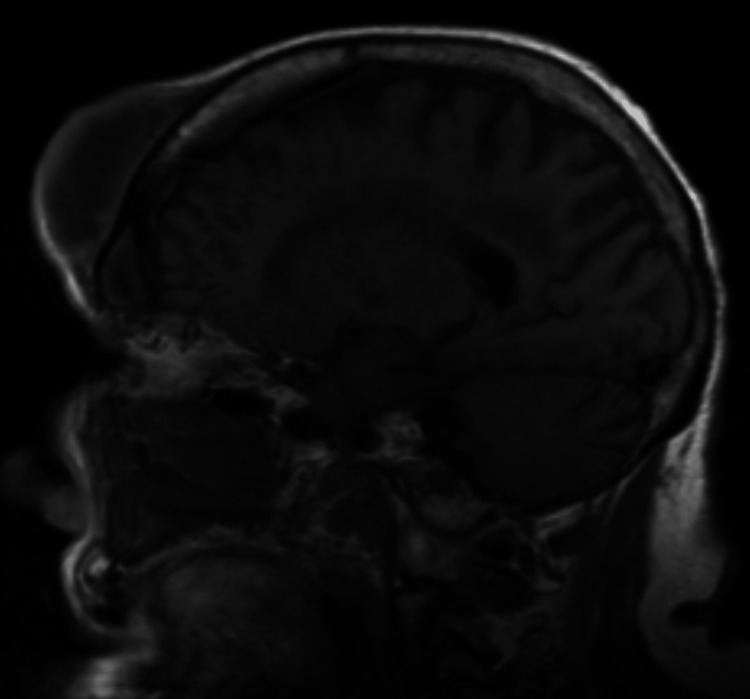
MRI of the brain showing bifrontal, maxillary, and ethmoid sinusitis. Bony erosion of the frontal calvarium extending into the bifrontal extra-axial collection. The findings are suggestive of abscess and frontal osteomyelitis with sinusitis.
